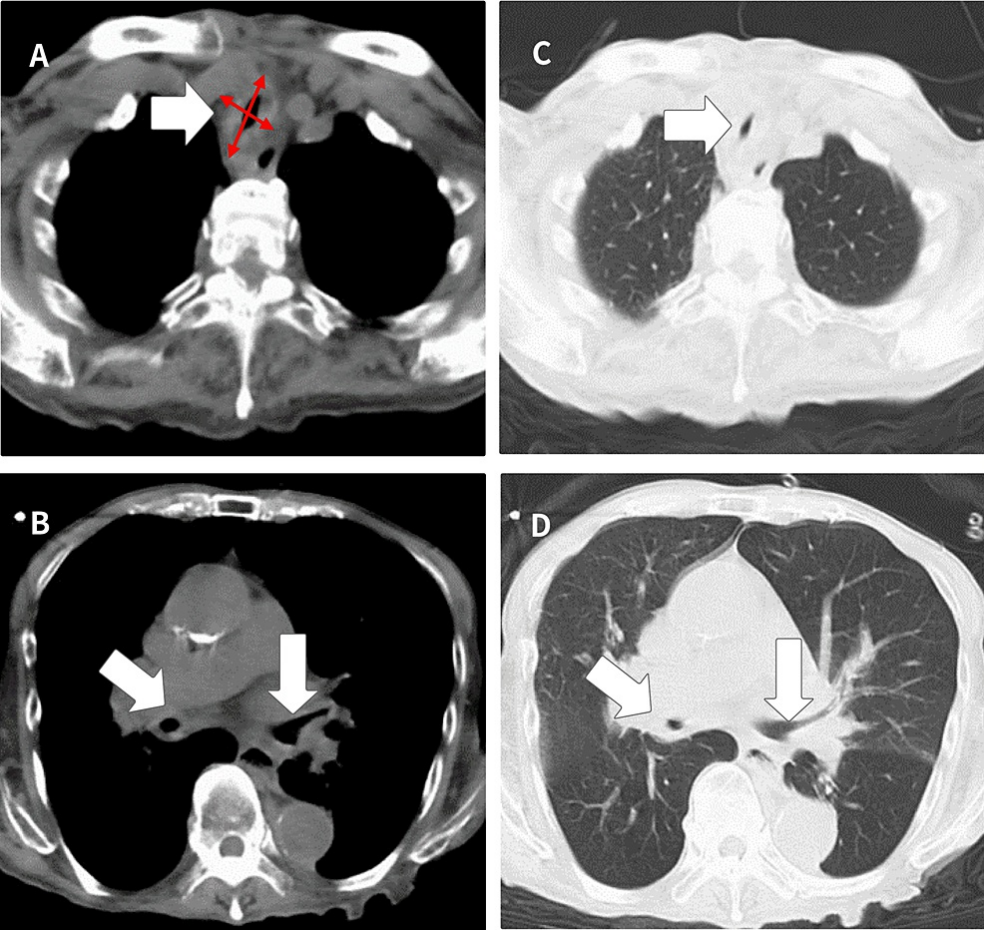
CT: (A and B): Soft tissue imaging conditions and (C and D): lung field imaging conditions. As indicated by the white arrows, there was a thickening of the bronchial wall from the central to the peripheral region. On the central side (A, C), the anteroposterior diameter is longer than the transverse diameter (red arrows) due to the lack of wall thickening in the membranous part of the posterior surface, resulting in a saber-sheath-like morphology. No morphological changes were seen in the bronchi after the bifurcation because of the absence of the membranous part of the posterior wall.
